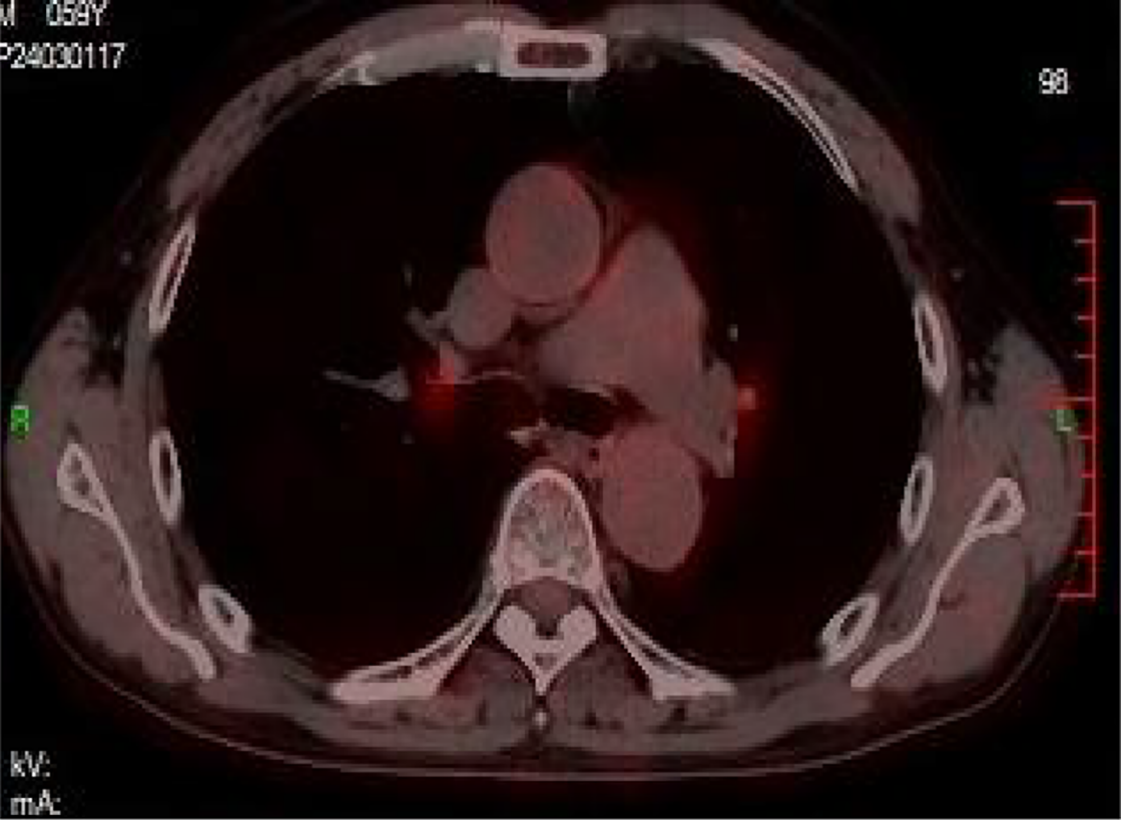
PET-CT: No abnormal hypermetabolic foci were seen in the pulmonary artery tract.
